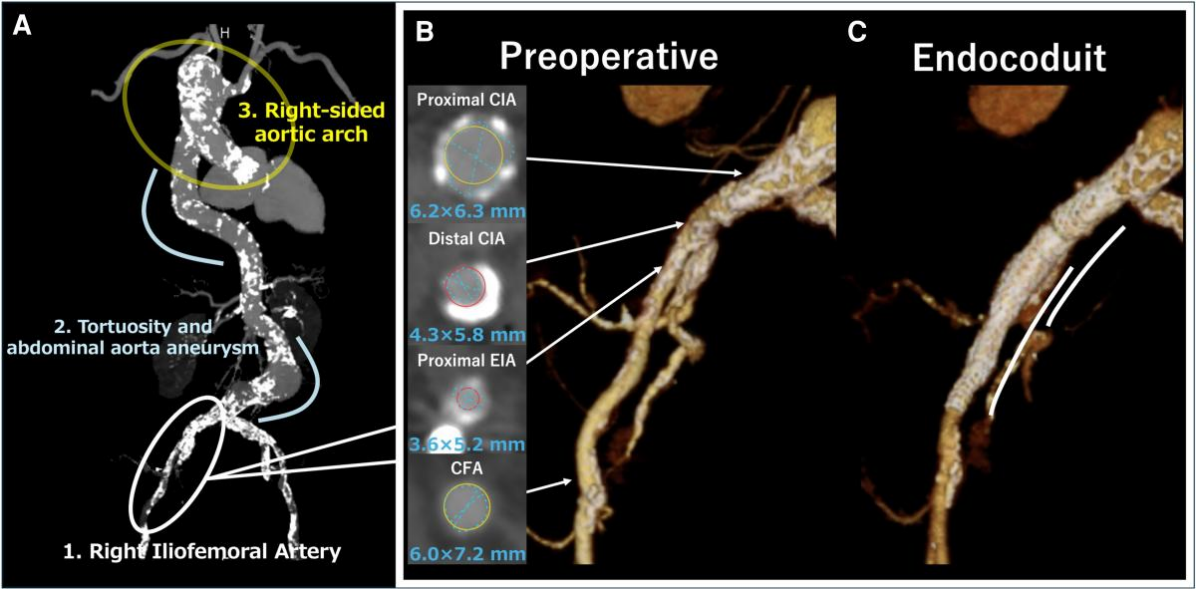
(*A*) Computed tomography angiography showing three major anatomical considerations for TAV-in-TAV. (*B*) Preoperative image of the iliofemoral arterial anatomy. Cross-sectional images showing measurements at various key points. (*C*) Post-operative image after the endoconduit procedure.
